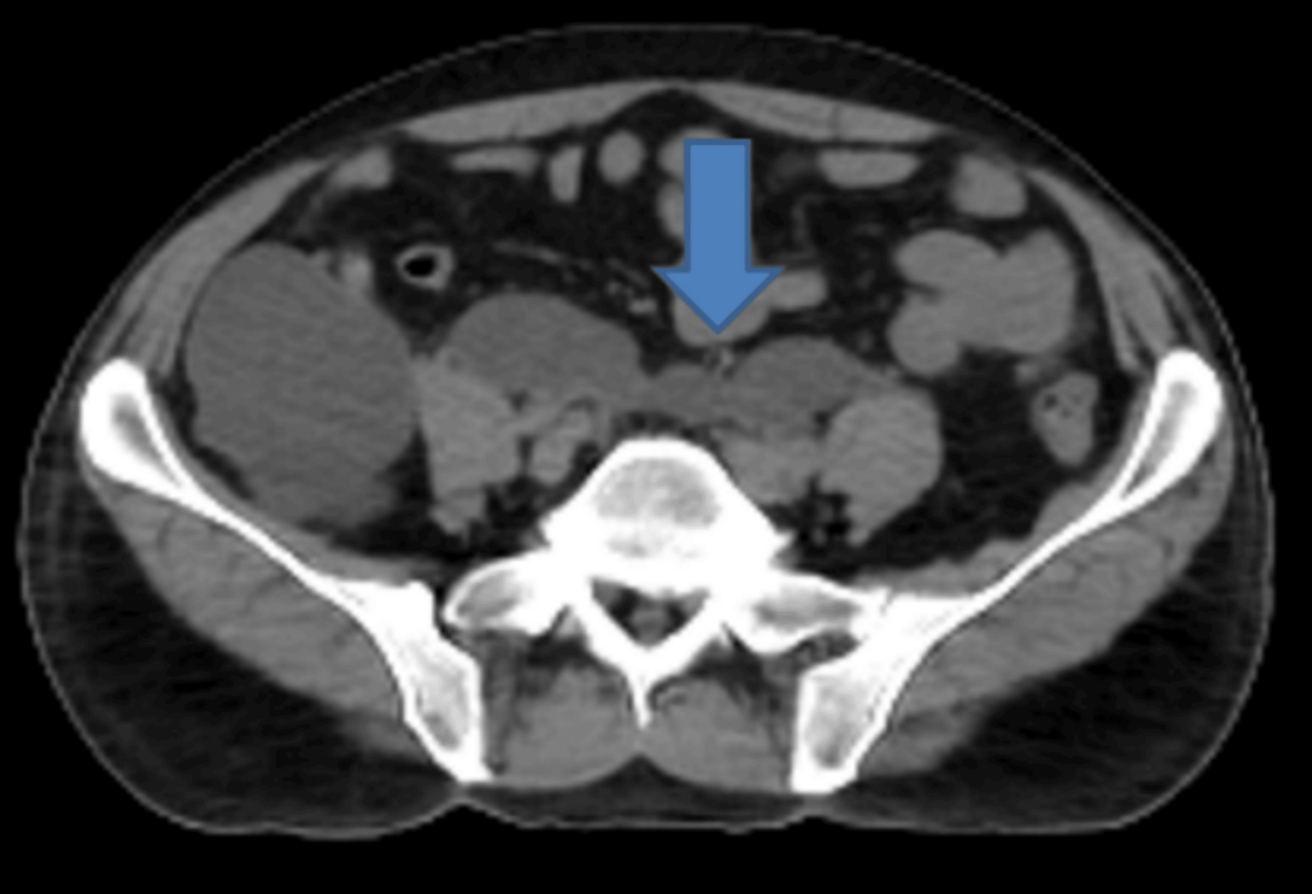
Non-contrast CT of the abdomen (axial view) showing an extension of the right lumbar region fluid collection across the midline (blue arrow) into the left lumbar region.
